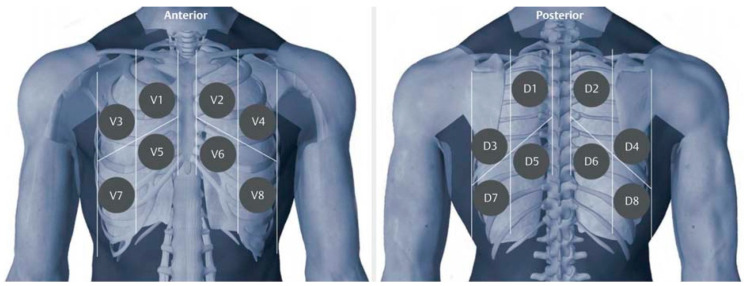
Scanning zones for intercostal lung ultrasound. Scanning zones for imaging data generation to assess ultrasound changes.
